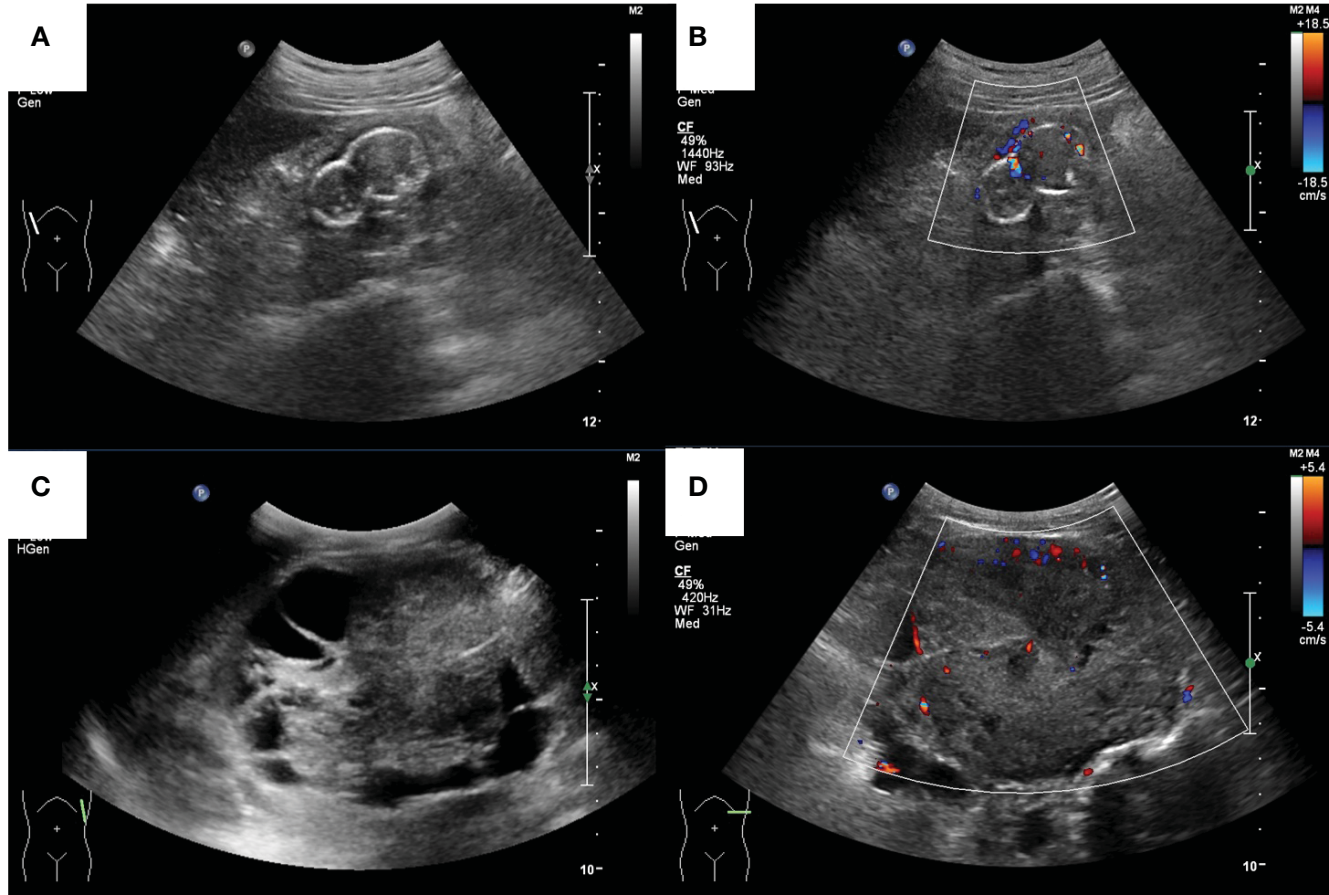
The ultrasound images of a 39-year-old male (case 2) and a 17-year-old male (case 4). (A) Grey-scale and (B) color Doppler sonograms of the mass in the right kidney in case 2. (C) Grey-scale and (D) color Doppler sonograms of the mass in the left kidney in case 4.
